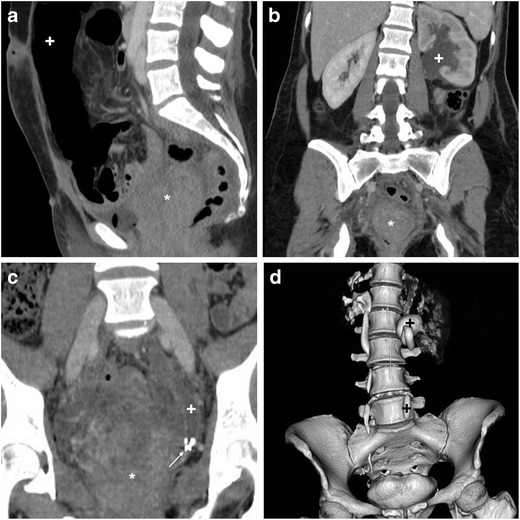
Ligation of the left ureter manifesting 24 h after AH. Unenhanced CT (A) showed a sizeable vaginal vault haematoma (*) and residual pneumoperitoneum (+) as expected findings. After intravenous contrast, the left kidney showed hydronephrosis (+) and impaired nephrogram compared to contralateral side, caused by abrupt stricture of the distal ureter (+ in C) in the site of surgical clips (thin arrow in C). Surgical reoperation including clip removal was required to manage the obstruction
